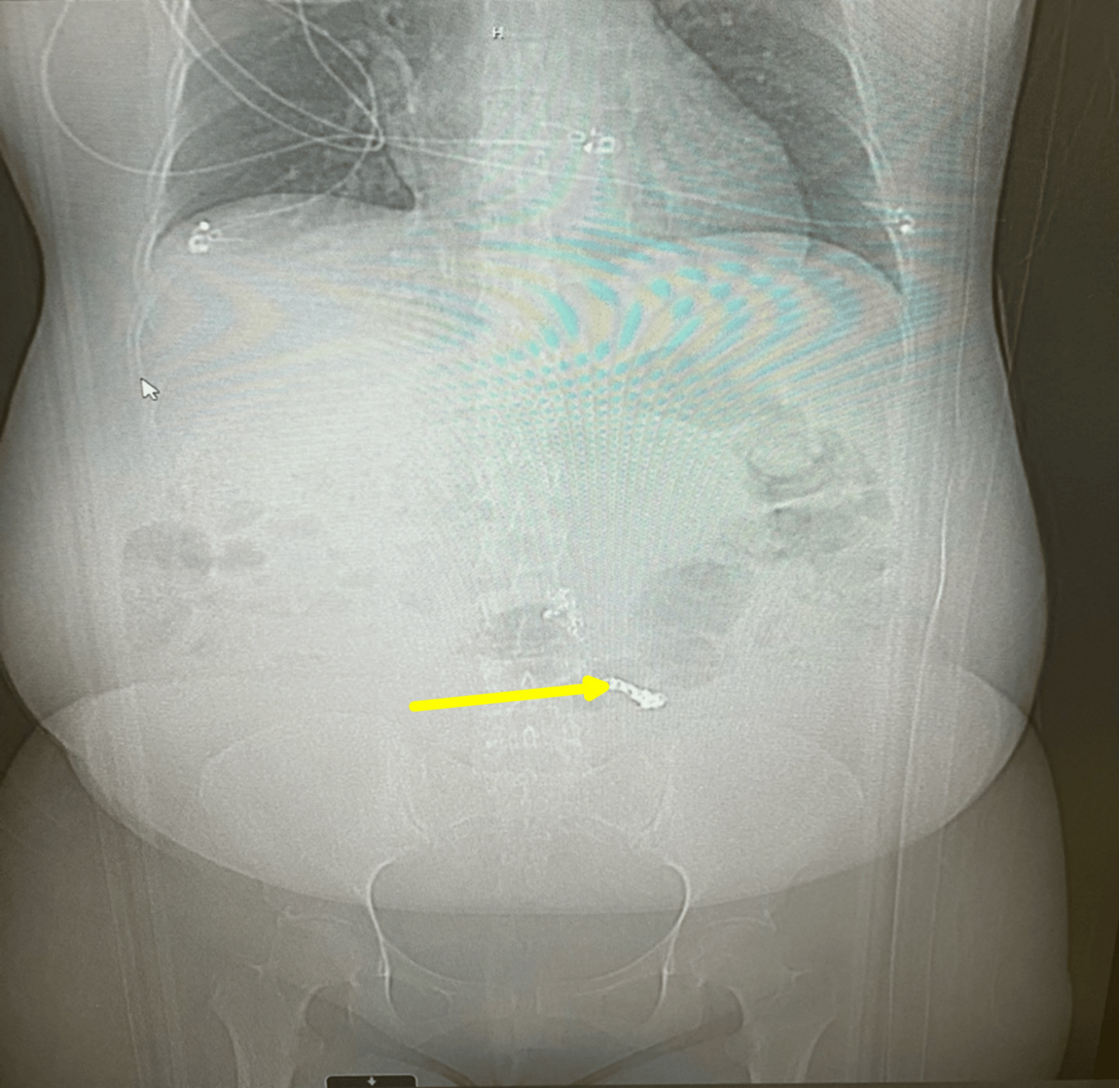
Superselective embolization using Ruby coils (Penumbra Inc.) and Gelfoam slurry (Pfizer Inc.), achieving complete occlusion. Abdominal X-ray demonstrates coil position in aberrant artery.
